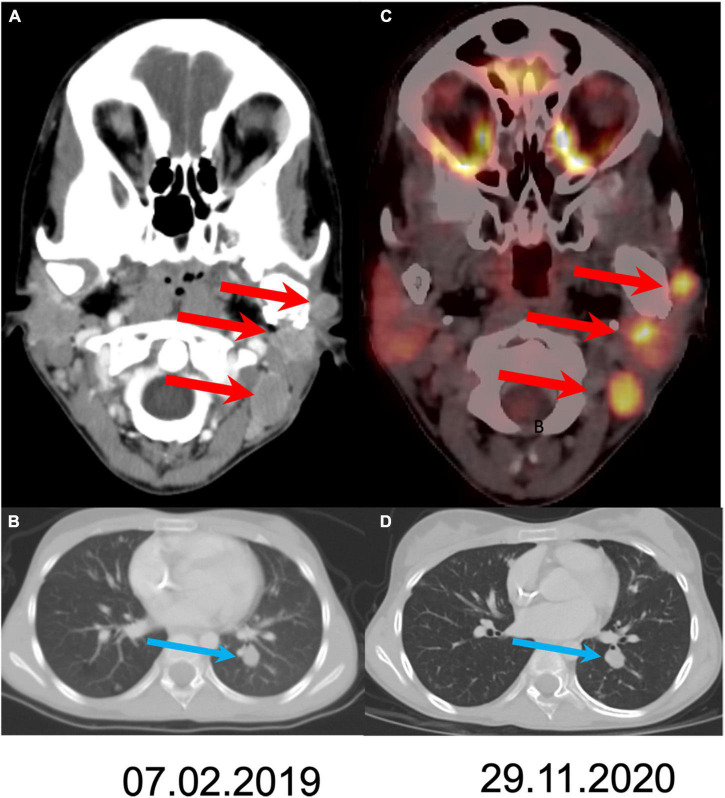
Comparison of disease extent at the initiation of treatment and after 21 months of follow-up. Axial CT slides at staging (A,B) show three metastases (arrows) in the left neck and lung spread. Follow up after 21 months shows stable disease both in the neck (C; fused PET/CT axial slide) and in the lung (D).
